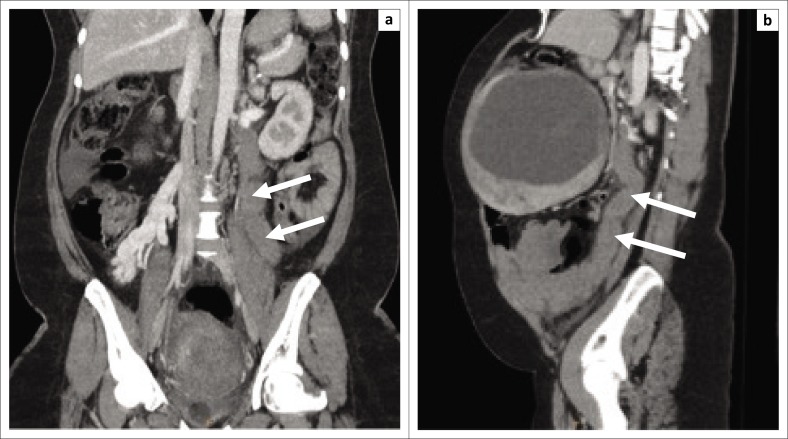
Coronal (a) and sagittal (b) portal venous computed tomography demonstrates tubular non-enhancing serpiginous structures corresponding to thrombosed left ovarian and parametrial veins (arrows).
